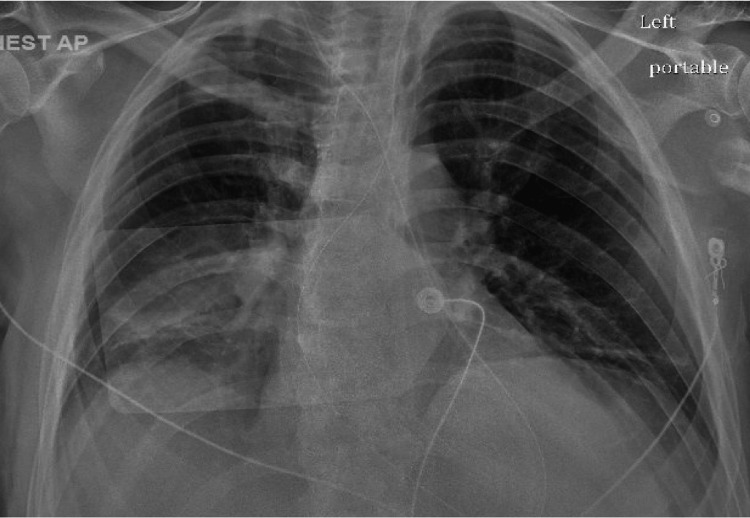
Initial chest x-ray, neg for any acute pathology.
